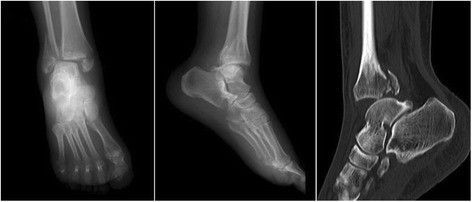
Preoperative X-ray and CT images showed unstable trimalleolar fractures involving posterior ankle comminuted fracture
